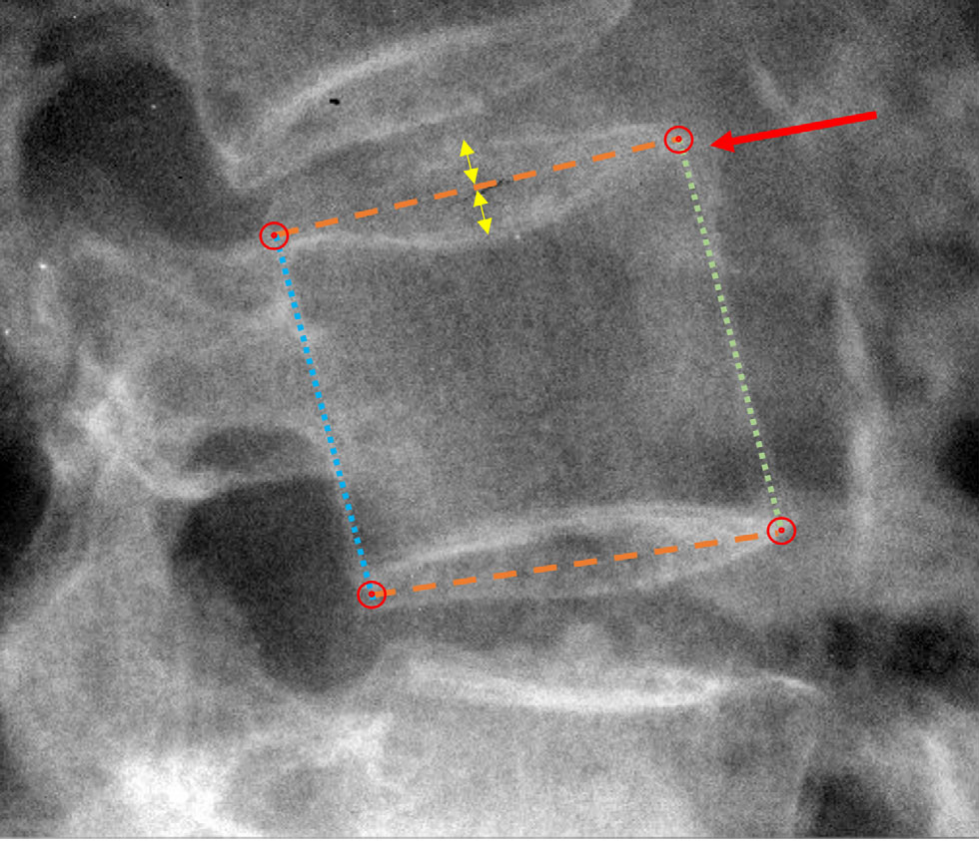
Details of anatomic landmark placement. Dashed lines show assumed midsagittal plane of superior and inferior endplates, identified as bisecting the radiographic shadows of the left and right sides of the endplates (yellow arrows). The red circles show the four landmarks used to measure vertebral body morphometry. The red arrow points to an anterior osteophyte that is ignored. The dotted lines show the anterior and posterior vertebral body heights.
